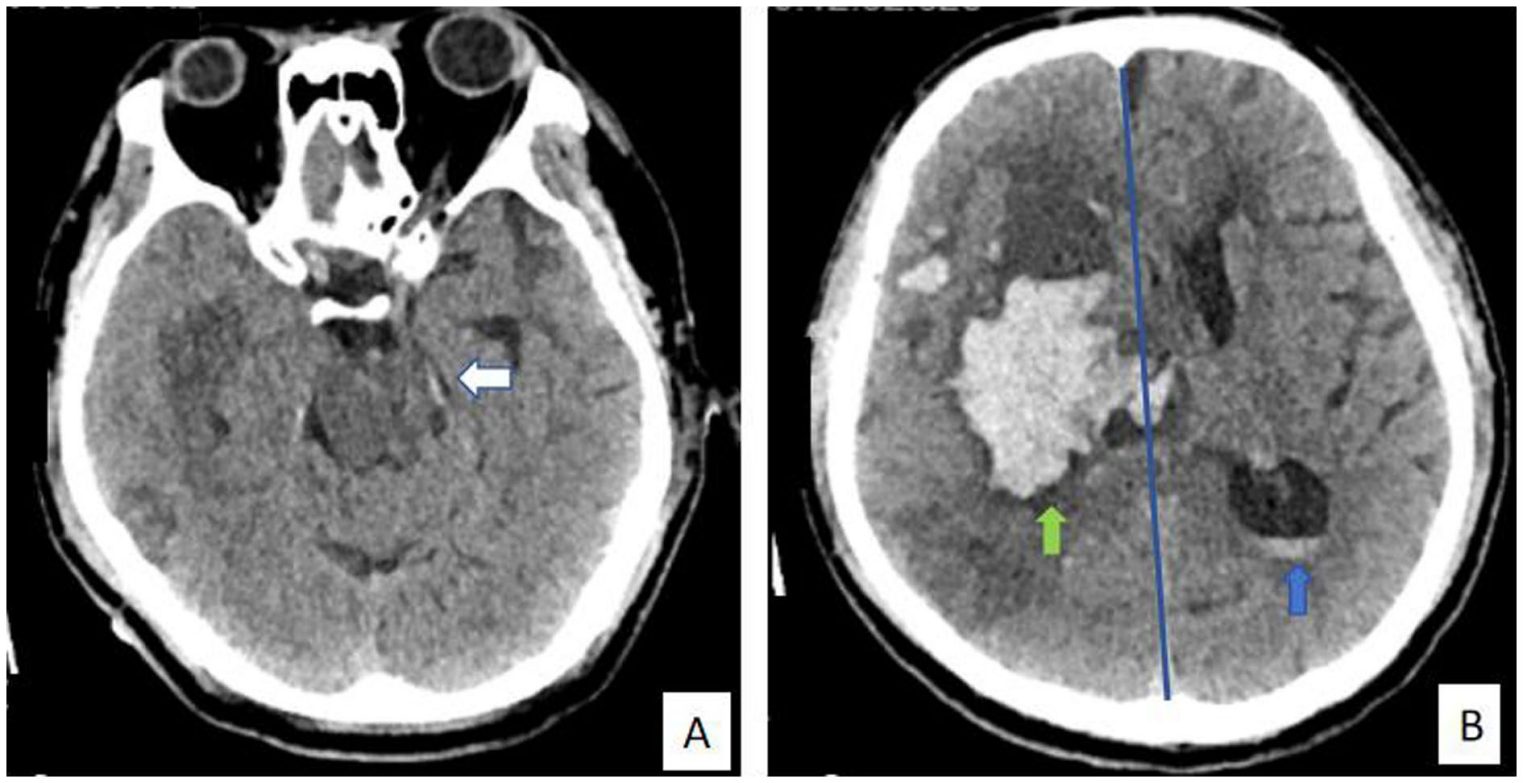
(A, B) A 66-year-old male patient with cerebral hemorrhage was admitted to the hospital and underwent surgical treatment. The final prognosis was not good. Rotterdam CT score are as follows: basal cisterna status: one point for compression (white arrow); midline displacement: one point for a shift >5 mm (blue line); ventricular hemorrhage: one point for presence (blue arrow); and space-occupying lesions, such as hematomas or contusions: one point for presence (green arrow). The total score plus one resulted in five points, which was used to predict prognosis.
